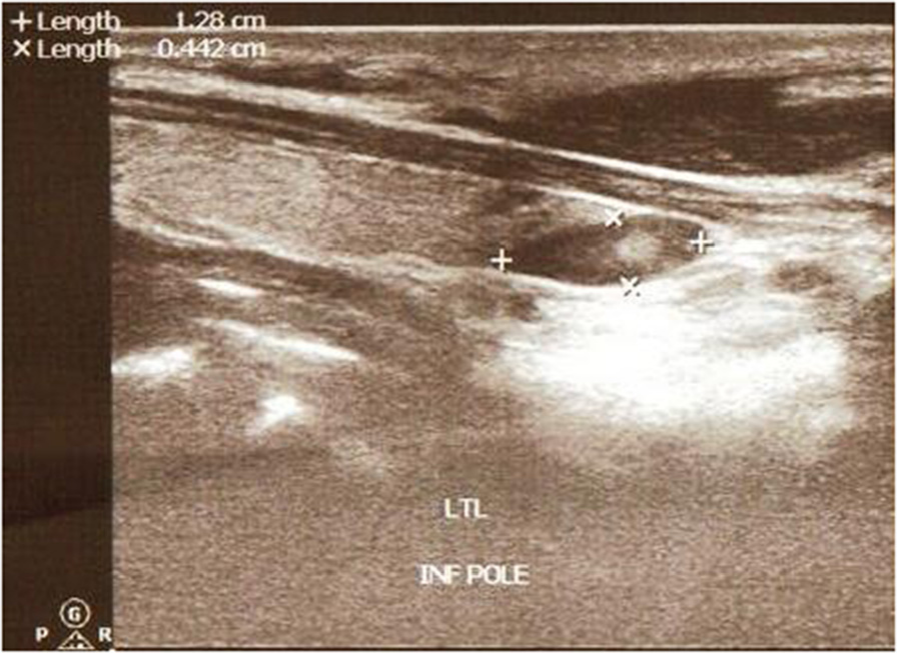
A neck ultrasound image showing a hypoechoic lesion (1.23 cm x 0.442 cm) close to the lower pole of thyroid gland.
